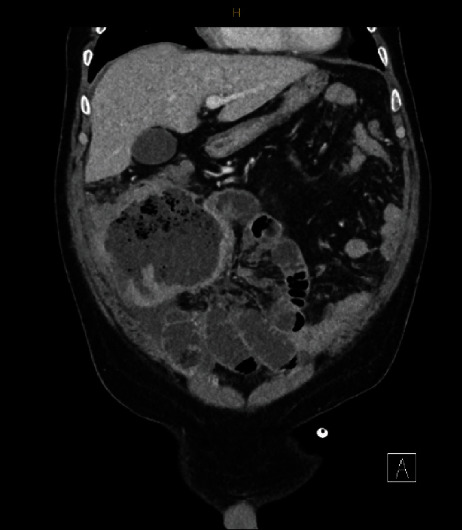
Pre-operative coronal CT images demonstrating the faecaloma.
